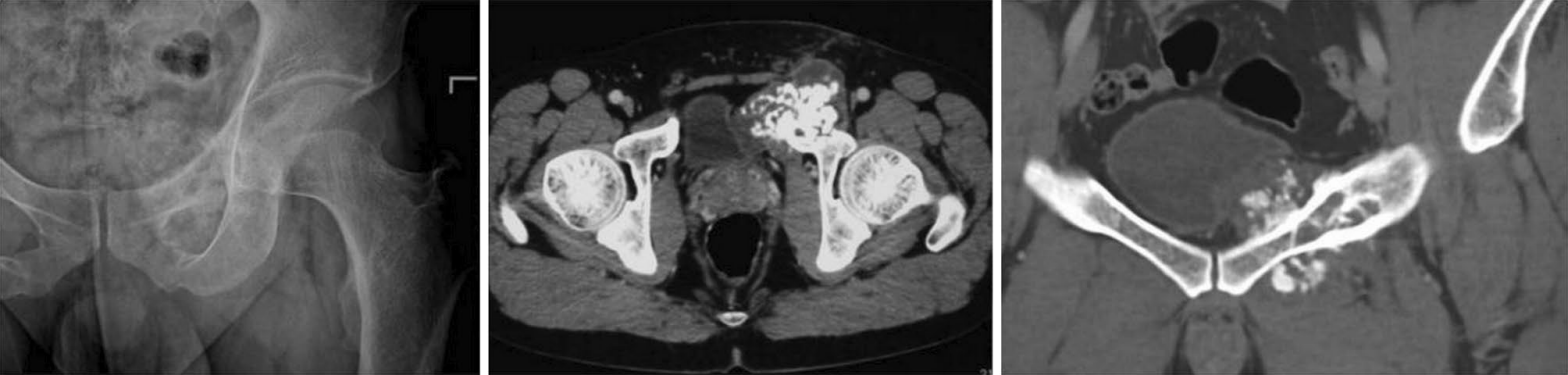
The preoperative radiography and CT of a 33 years male with chondrosarcoma of left anterior column of acetabulum.
